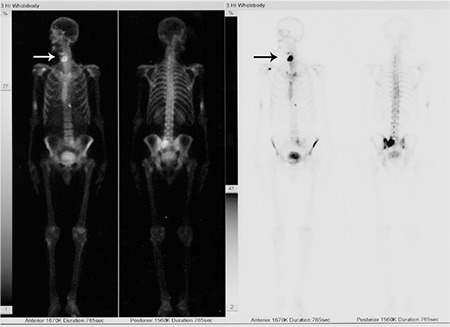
Bone scintigraphy shows accumulation of Tc-99m at the two sites of the anterior neck (arrows). Note also the accumulation of Tc-99m in the left iliac bone, sacrum and the right proximal humerus.
